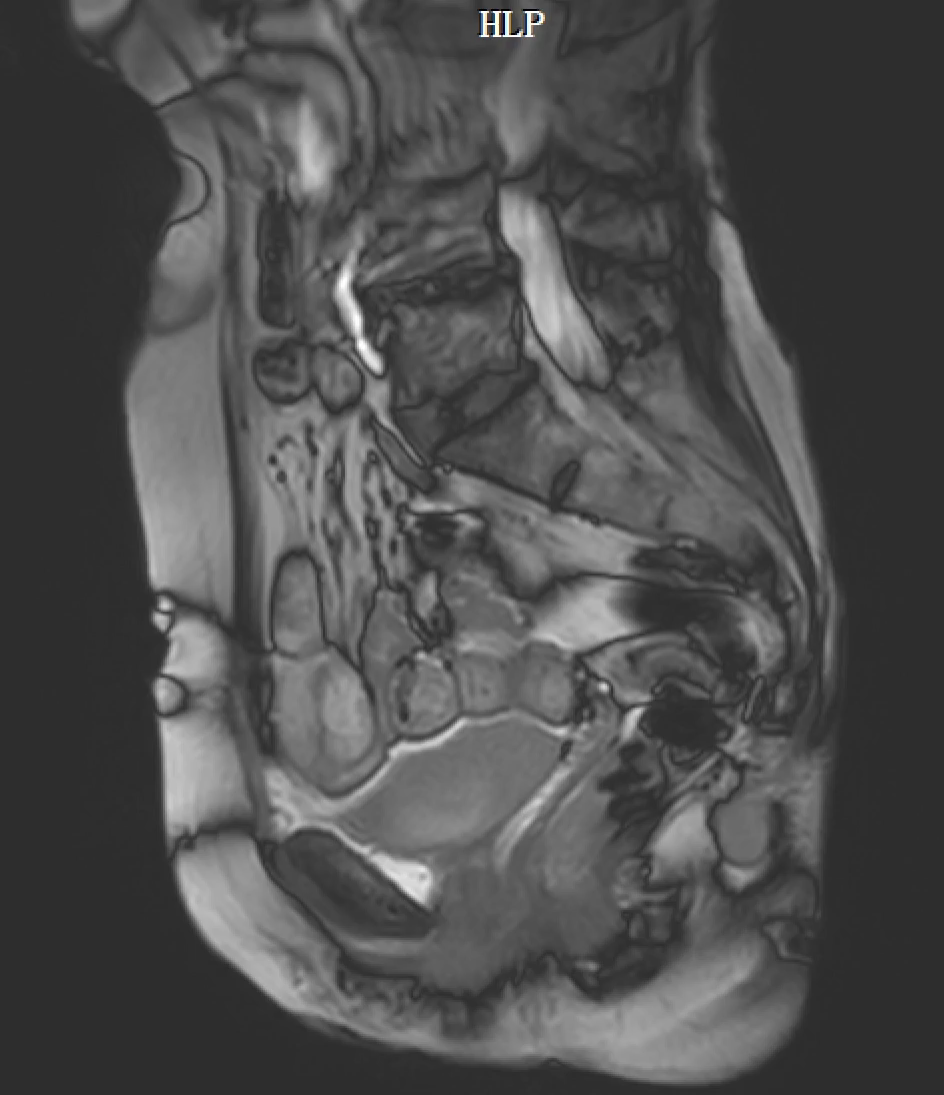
Computed tomography image of the older female participant. HLP: head left posterior.
